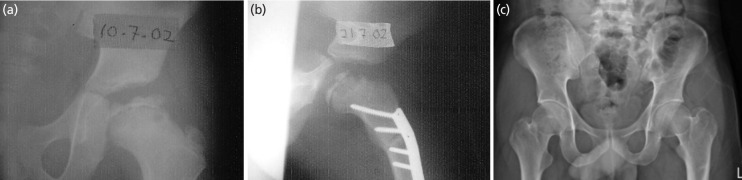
The series of radiographs belongs to patient no.2. (a) The presentation at the age of 9 showed fragmentation phase with Herring B. (b) The immediate post-surgery showed good containment. (c) The hip at the age of 28 years old showed Stulberg I.
